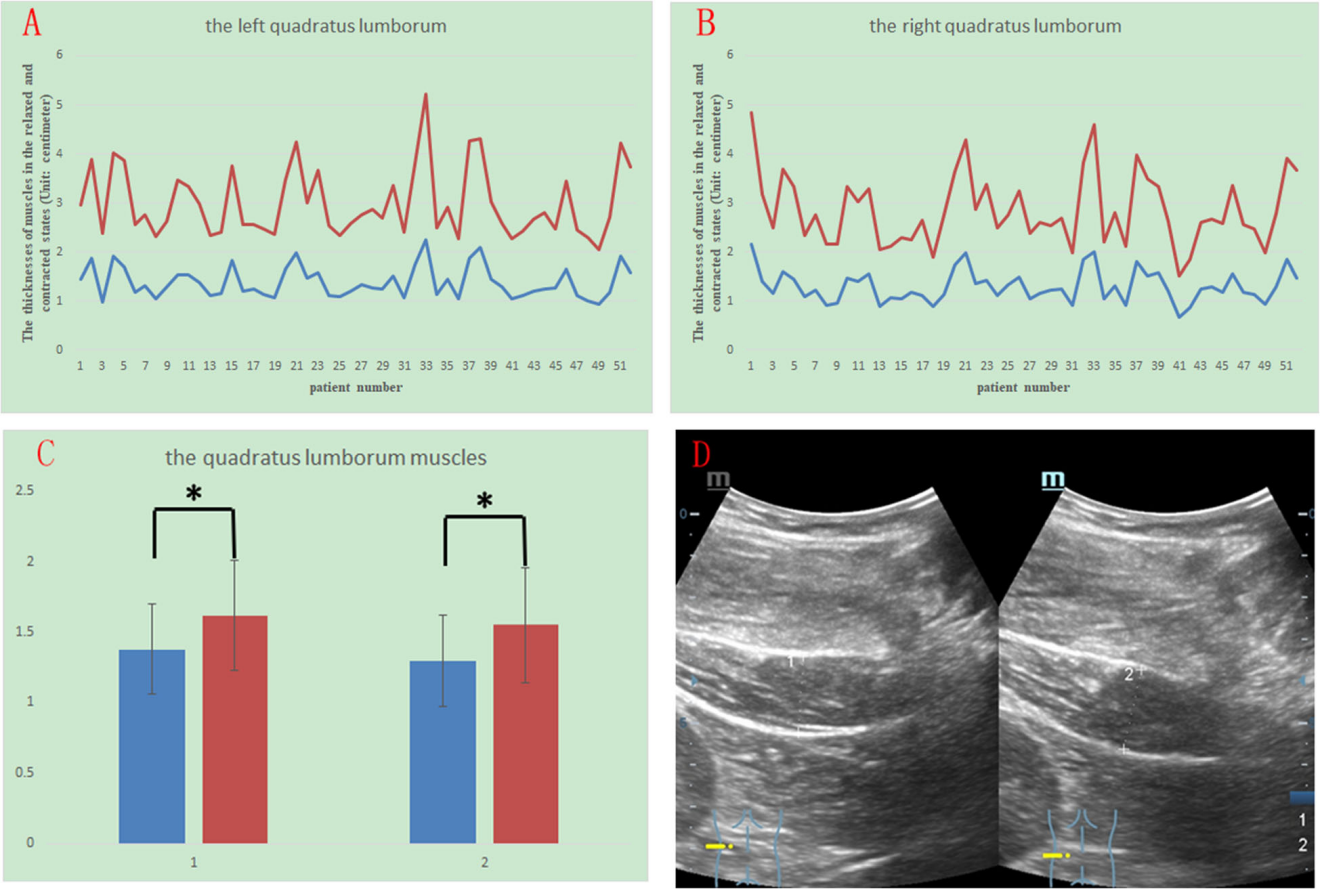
The thicknesses of the left (Fig. 3 A) and right (Fig. 3B) quadratus lumborum muscles in the resting (blue line) and contracted conditions (red line). Comparison of the thicknesses in the resting (blue column) and contracted conditions (red column). Group 1 is right, and group 2 is left (Fig. 3 C). The sonogram of the quadratus lumborum: left shows the relaxed state, and right shows the contracted state (Fig. 3D)
